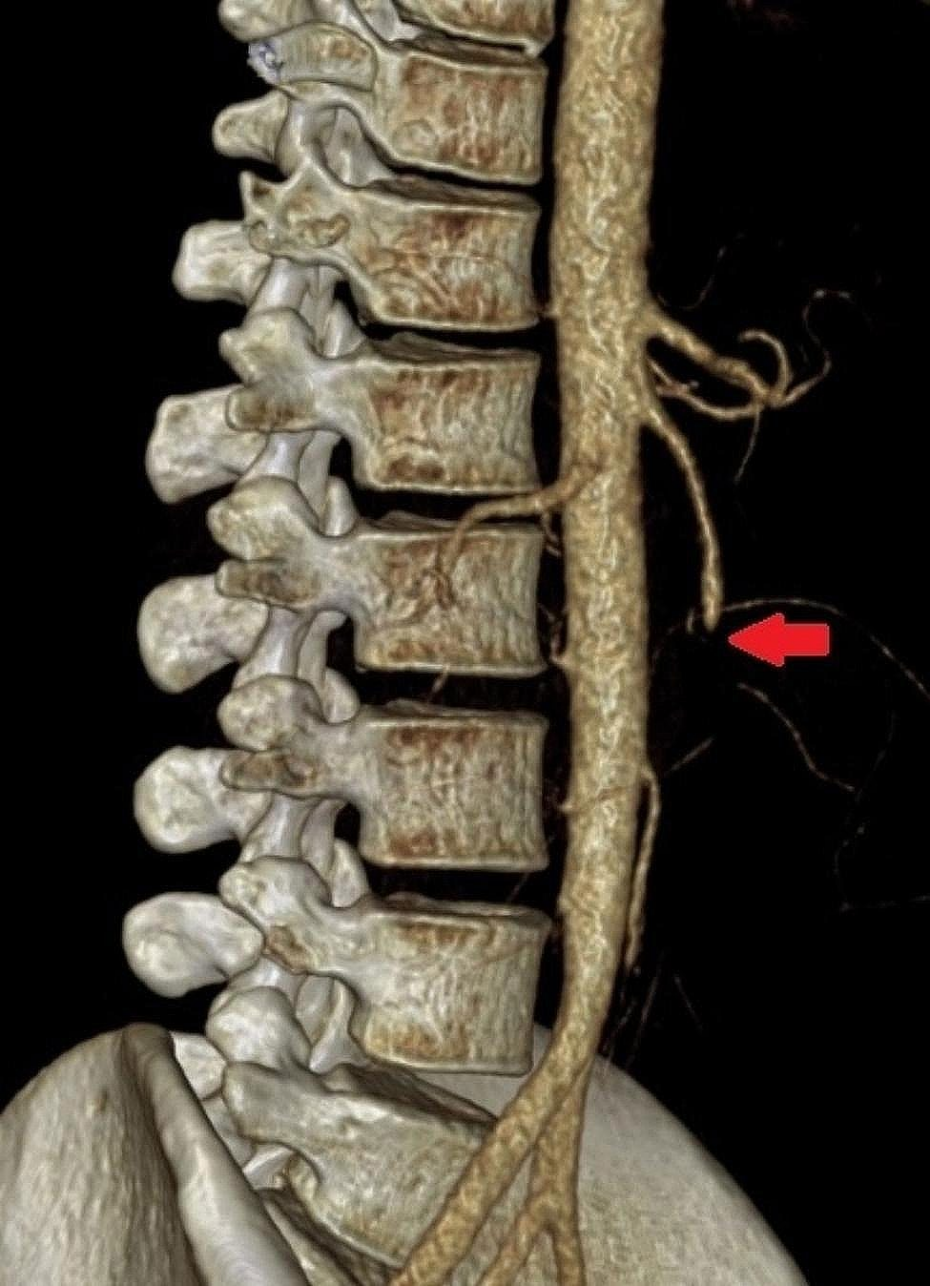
3D reconstruction of the CTA showing SMA occlusion (Reference: Semmelweis University – Medical Imaging Centre
